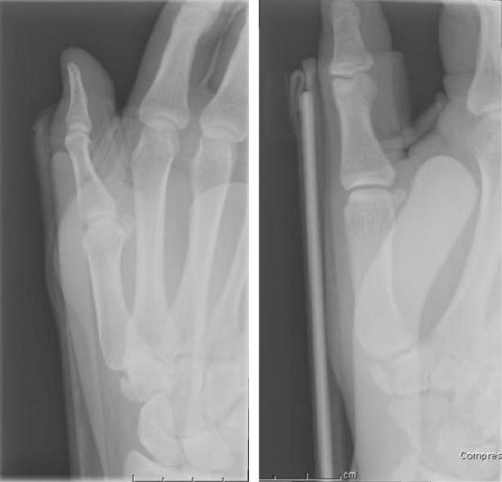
Plain radiograph of right hand post closed reduction of carpometacarpal dislocation.
